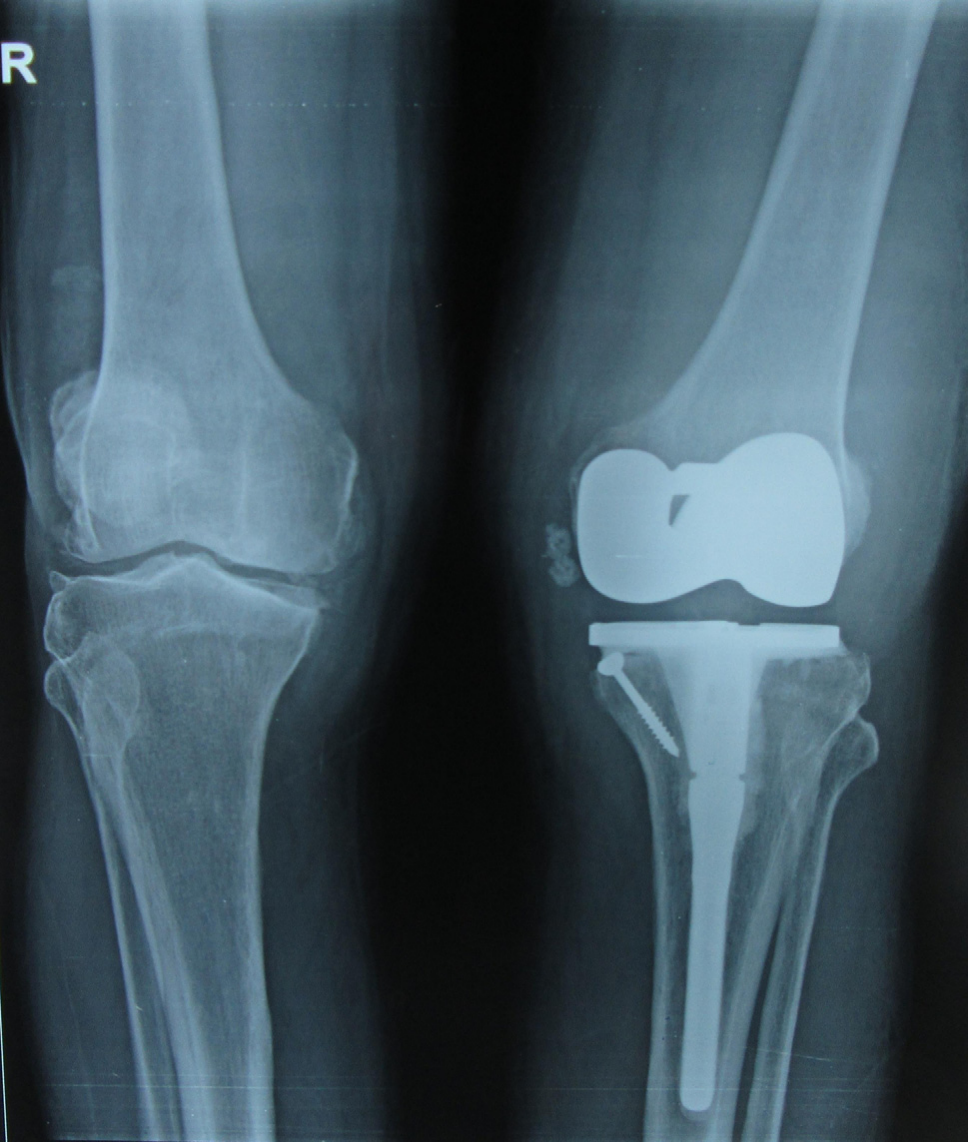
A standing anteroposterior radiograph of the left knee shows a well-fixed, well-aligned, total knee prosthesis with cemented tibial stem and a malleolar screw in the medial aspect.
